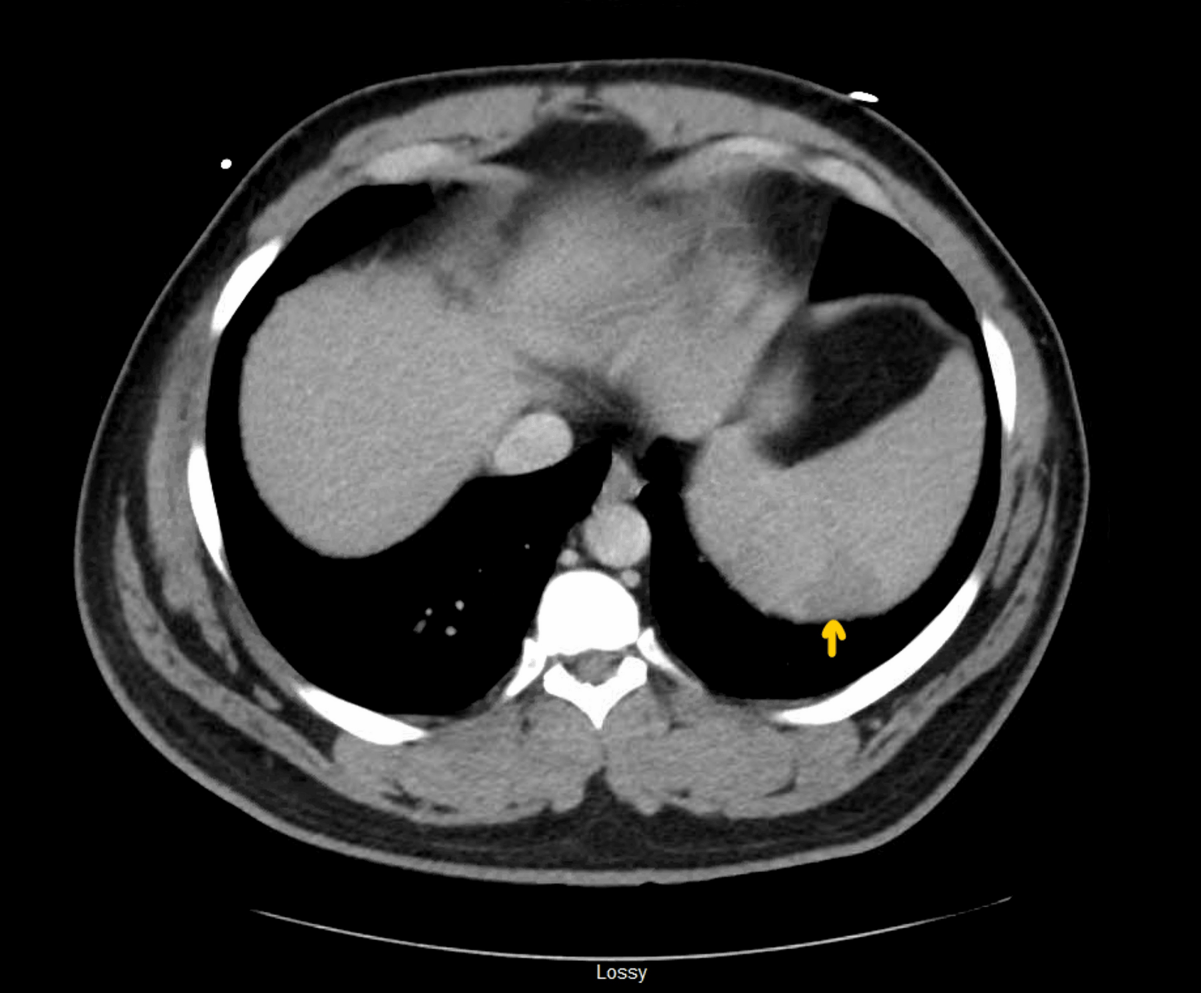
CT scan abdomen with IV contrast revealed splenomegaly with a wedge-shaped infarct
